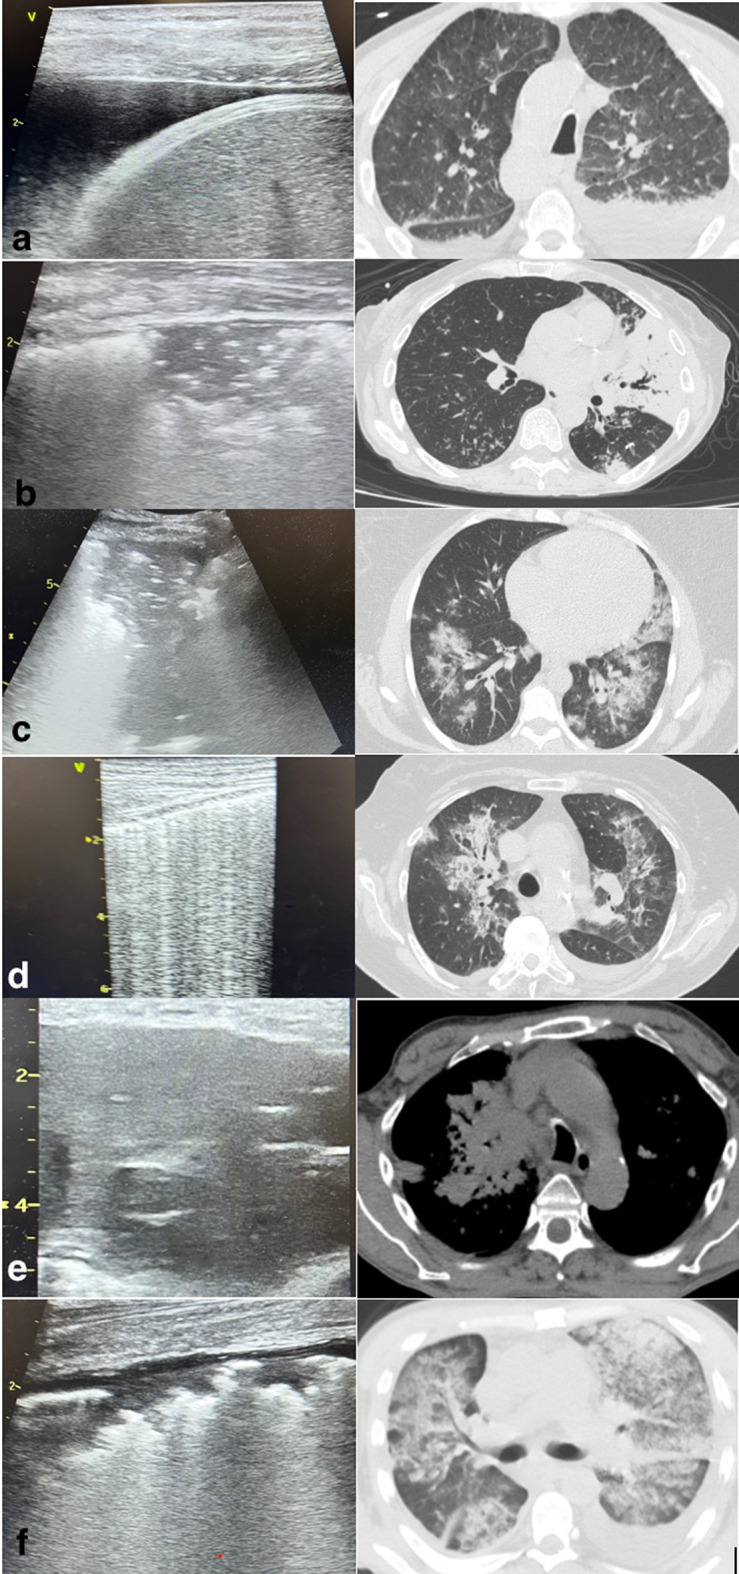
Images of LUS findings compared with thoracic CT scans. a) Pleural effusion; b) Pneumonia; c) Bronchopneumonia; d) Acute pulmonary edema; e) Pleuropulmonary tumors; f) ARDS. (images from personal archive)
